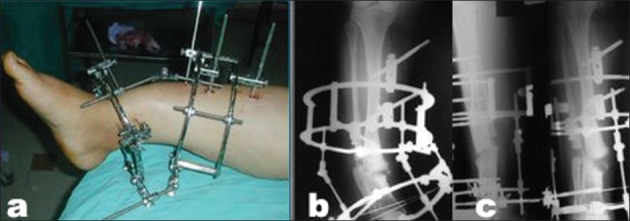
(a) Intraoperative clinical photograph shows the application of a preconstructed Ilizarov fixator and fibular osteotomy for closed distraction of the nonunion. (b, c) Postoperative radiographs show gradual deformity-correction.
